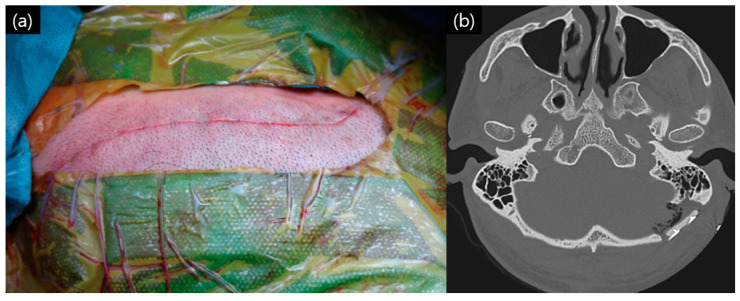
Completed closure: (a) operation site after subcutaneous layer suture; (b) immediate postoperative temporal bone computed tomography (CT) of the patient.
